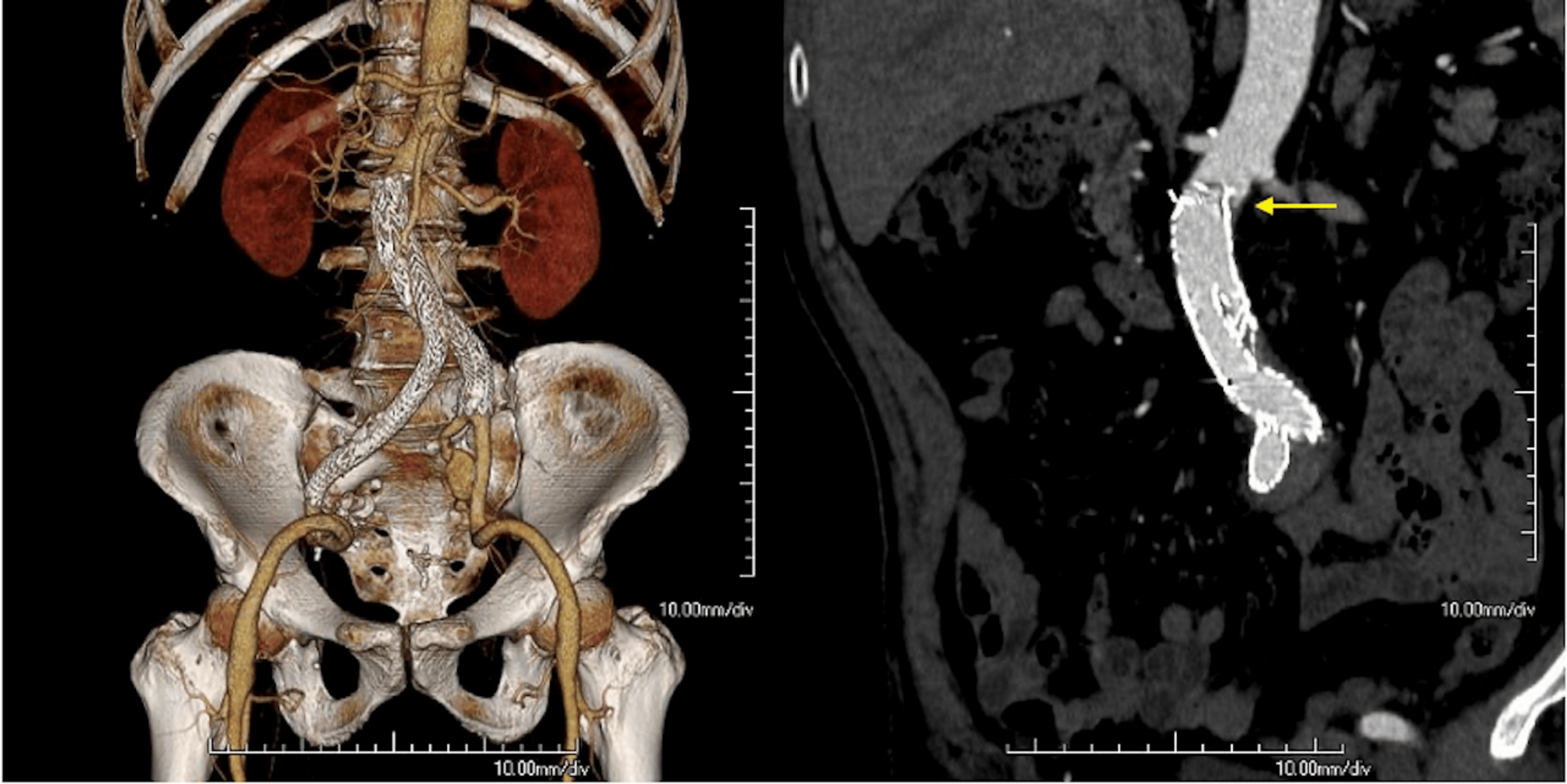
Volume-rendered reconstruction and coronal section of CTA six days after EVAR The arrow indicates a small bird-beak appearance, which was caused by slightly lower deployment of the endograft. CTA, CT angiography; EVAR, endovascular aortic aneurysm repair
